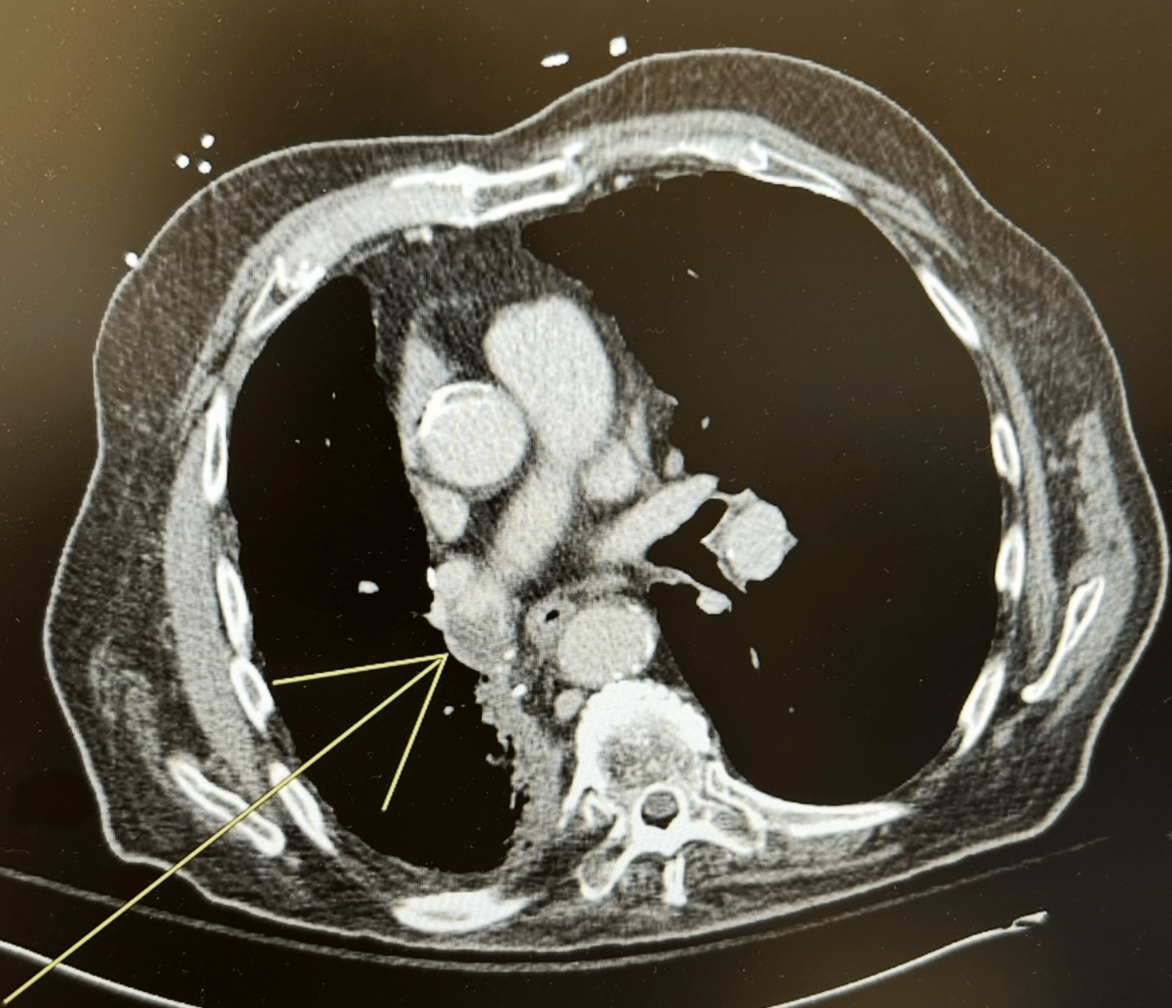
Thin-slice (1 mm) contrast-enhanced axial CT scan showing a concave intraluminal filling defect within the right pulmonary artery stump (yellow arrow). The thrombus measures approximately 15 mm in length by 8 mm in width.
